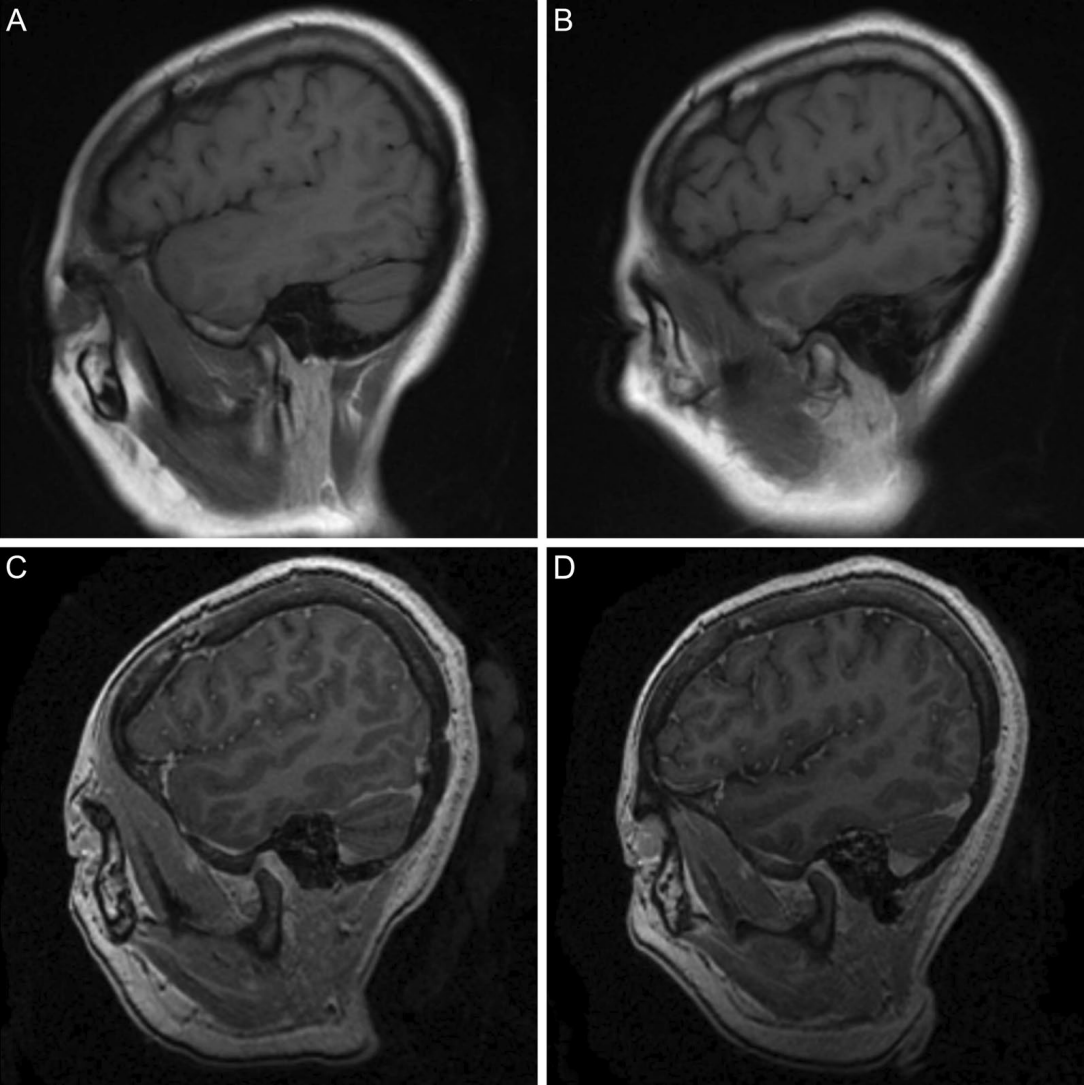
Selected T1-weighted (a, b) and contrast-enhanced three-dimensional fast spoiled gradient-echo (c, d) magnetic resonance imaging of the left (a, c) and right (b, d) temporomandibular joints demonstrating normal osseous anatomy and articular disc position
